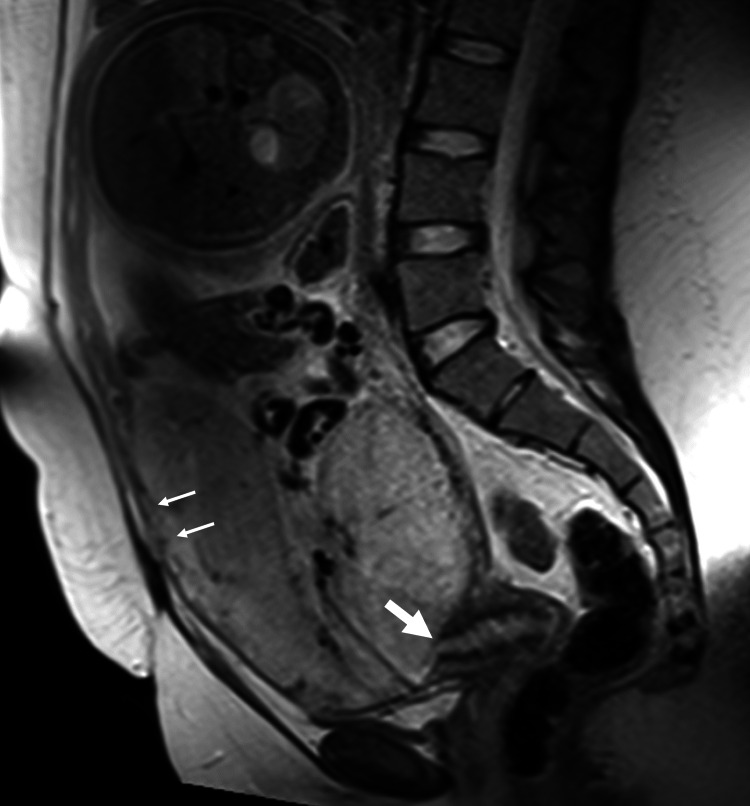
MRI Pelvis Sagittal view Placenta demonstrating increased anterior vascularity and a focal area of thinning at the myometrial interface, suggestive of placenta accreta. The internal os appears covered, but findings were more concerning for abnormal placental invasion.
